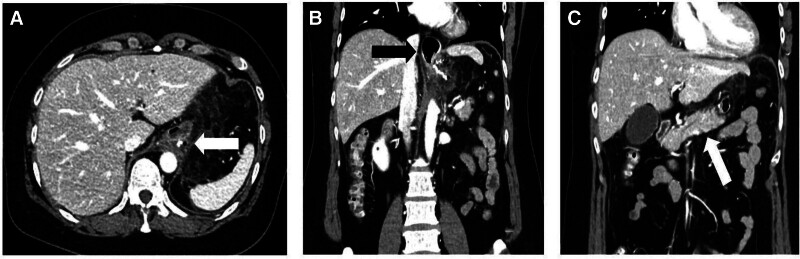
Abdominal CT performed during the initial presentation to the emergency department. (A) The white arrow indicates an air bubble with intra-abdominal fluid collection. (B) The black arrow indicates the dilatation of the upper gastric portion. (C) The white arrow indicates peritoneal wall enhancement. CT = computed tomography.
